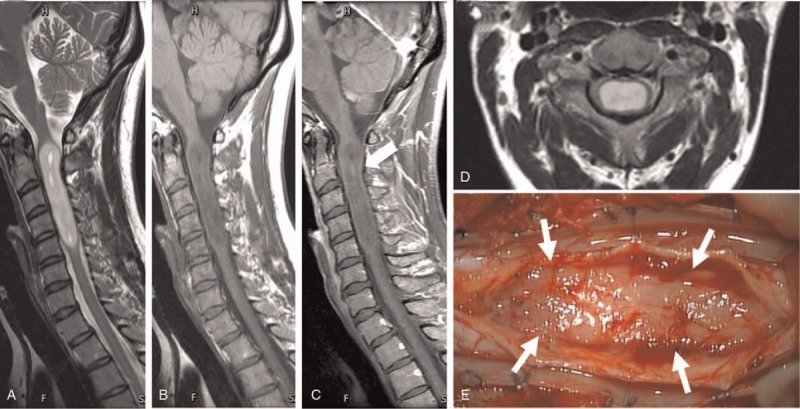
Magnetic resonance imaging (MRI) demonstrates the well-defined intramedullary tumor in the spinal cord from C1 to C5 level. (A and B) Sagittal images showing low intensity in T1-weight imaging, high intensity in T2 weight imaging. (C) Contrast-enhanced MRI showed a very slight enhancement at the C2 level (black arrow). (D) Axial image of T2-weighted MRI demonstrating lateralization of the tumor to right side. (E) Intraoperative picture following midline myelotomy is shown. Arrows indicate the tumor inside the spinal cord.
